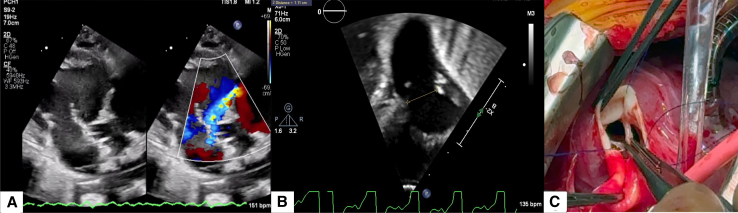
A, Preoperative echochardiography showing new-onset regurgitation with eccentric jet (Video 2). B, Preoperative echocardiography showing pulmonary annulus measuring 11 mm. C, Intraoperative finding of left pulmonic cusp free margin avulsion with lower forceps holding valve edge (Video 3).
